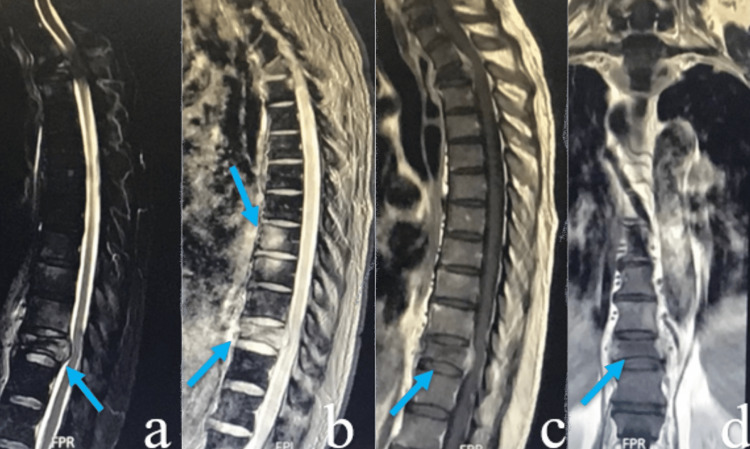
MRI demonstrating pathological collapse fracture in short tau inversion recovery (STIR) weighted (a), T1-weighted (b), T2-weighted (c, d) images of T2 and T11 vertebrae.
